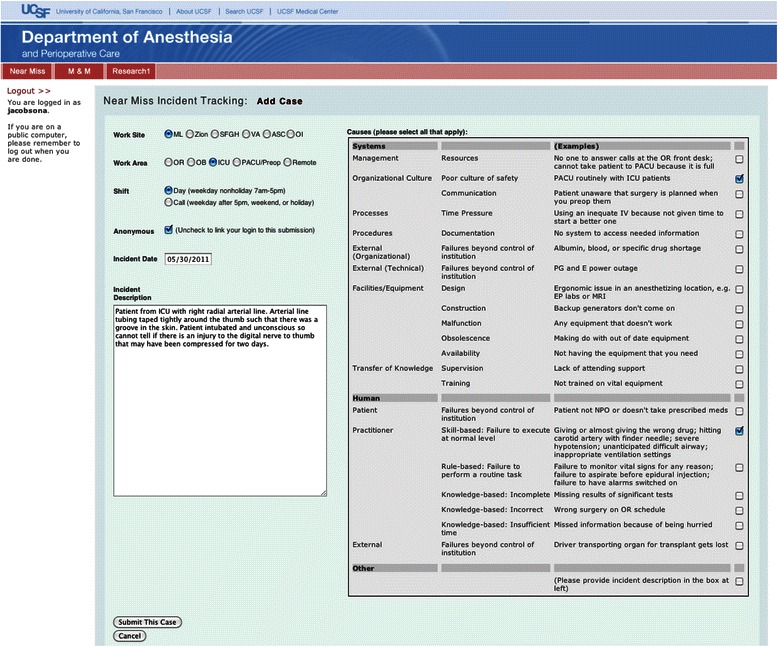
Near miss reporting system user interface. ML, Moffitt Long Hospital; Zion, Mount Zion Hospital; SFGH, San Francisco General Hospital; VA, San Francisco Veteran’s Affairs Hospital; ASC, Ambulatory Surgery Center; OI, Orthopedic Institute; OR, operating room; OB, obstetrical ward; ICU, intensive care unit; PACU/Preop, Post-operative and pre-operative care units; IV, intravenous line; PG and E, Pacific Gas and Electric; EP, electrophysiology; MRI, magnetic resonance imaging; NPO, nil per os (nothing by mouth)
